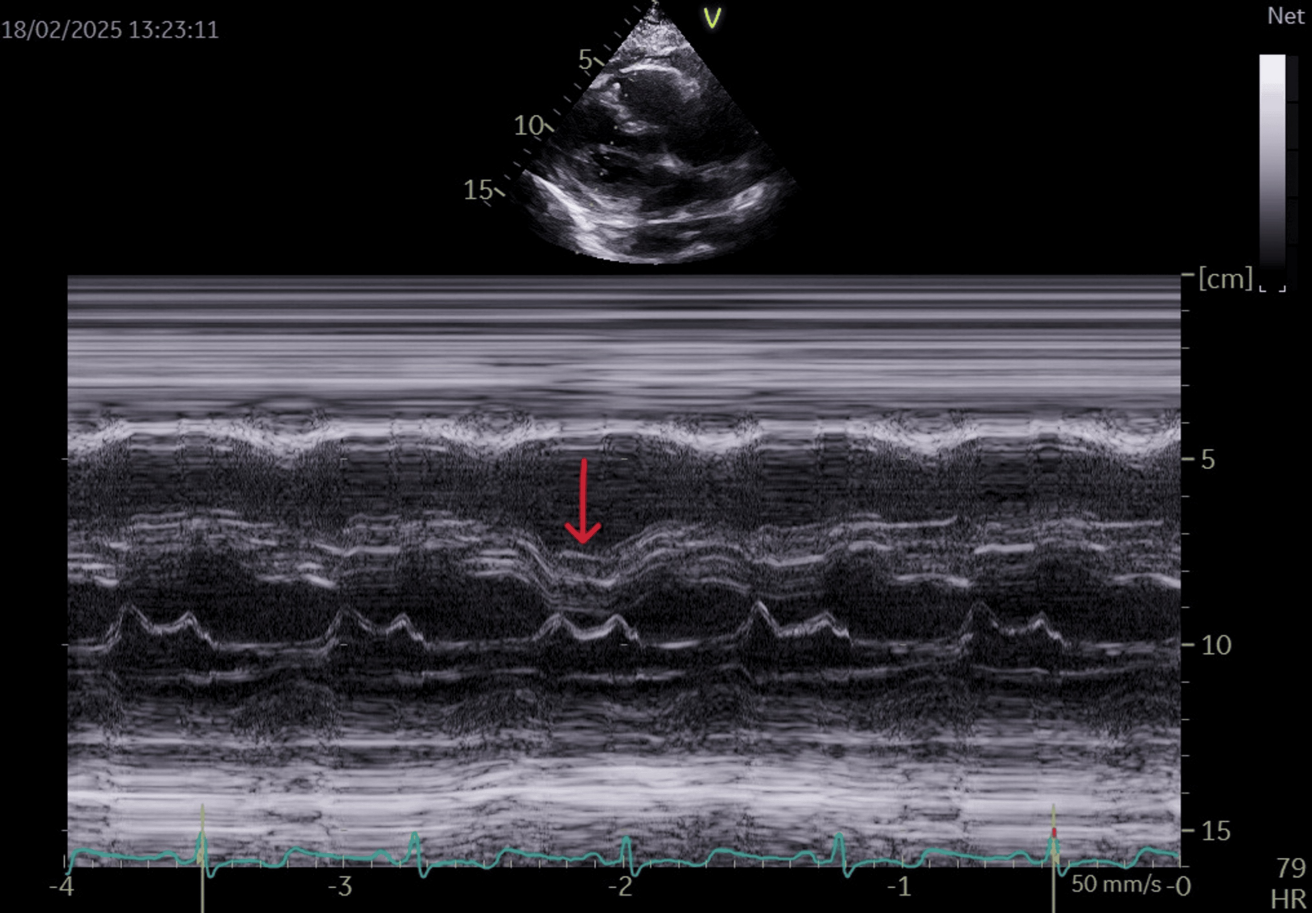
An M-mode echocardiographic image in the parasternal long-axis view shows diastolic septal bounce (arrow), with paradoxical motion of the interventricular septum toward the left ventricle during inspiration, a sign of right-left ventricular interdependence
